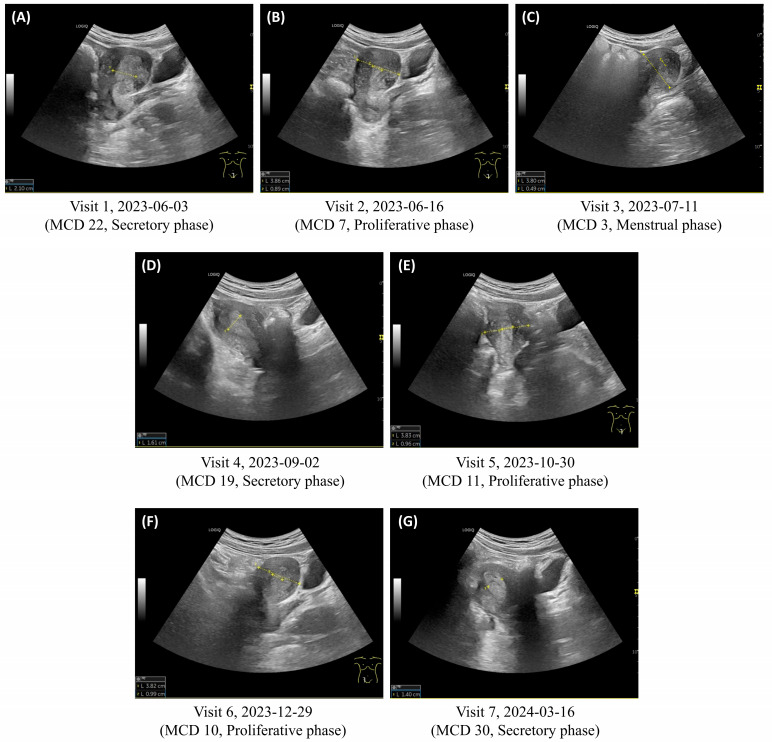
Transabdominal ultrasound images showing endometrial thickness measurements. Normal ranges by menstrual cycle phase are proliferative phase (0.4–0.8 cm), secretory phase (0.7–1.4 cm), and menstrual phase (0.1–0.4 cm). (A) Visit 1: endometrium measuring 2.10 cm in the secretory phase, exceeding the normal range. (B) Visit 2: 0.89 cm in the proliferative phase, exceeding the normal range. (C) Visit 3: 0.49 cm during the menstrual phase, exceeding the normal range. (D) Visit 4: 1.61 cm in the secretory phase, exceeding the normal range. (E) Visit 5: 0.96 cm in the proliferative phase, exceeding the normal range. (F) Visit 6: 0.99 cm in the proliferative phase, exceeding the normal range. (G) Visit 7: 1.40 cm in the secretory phase, within the normal range. MCD, menstrual cycle day.
